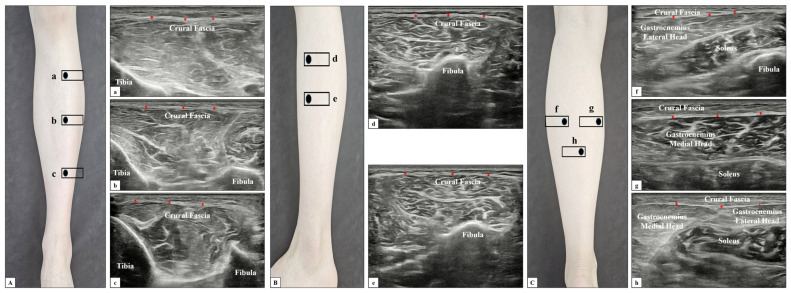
Ultrasound images of crural fascia thickness in the anterior (A), lateral (B), and posterior (C) compartments. (A) Probe at three positions in the anterior compartment (a–c), with corresponding ultrasound images indicating crural fascia measurements 1–3. (B) Probe at two positions in the lateral compartment (d,e), with corresponding ultrasound images indicating crural fascia measurements 1–2. (C) Probe at three positions in the posterior compartment (f–h), with corresponding ultrasound images indicating crural fascia measurements 1–3. Red arrows indicate the thickness of crural fascia.
